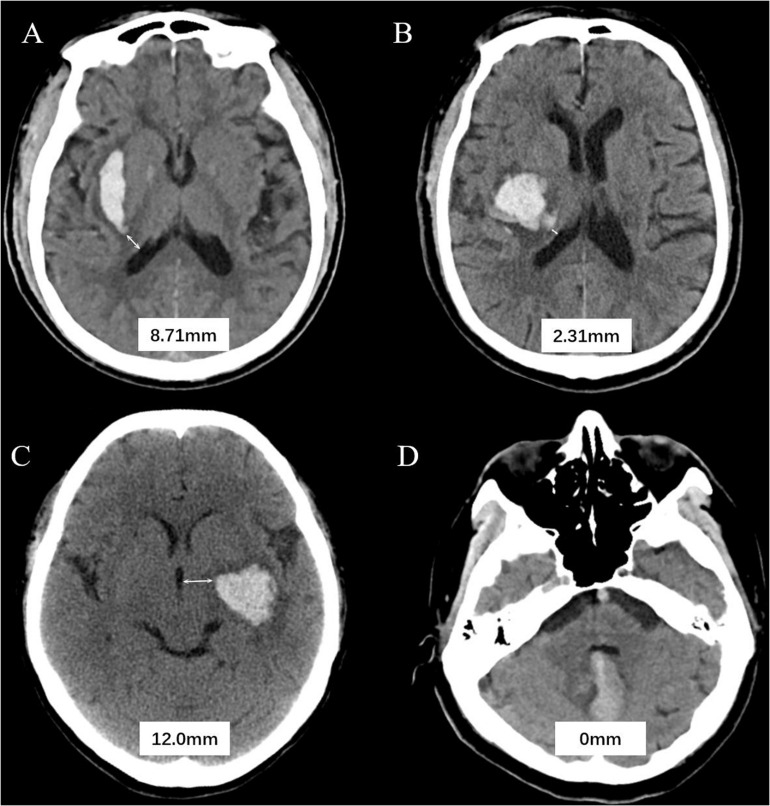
Graphic representation of the hematoma ventricle distance (HVD) on computed tomography. (A) A Baseline CT scan reveals a basal ganglia hematoma with HVD of 8.71 mm. (B) In patient B, the HVD is 2.31 mm. (C) A admission CT shows the distance between hematoma and the third ventricle is 2.0 mm. (D) Cerebellar hematoma reaches the border of ventricles without IVH extension.
